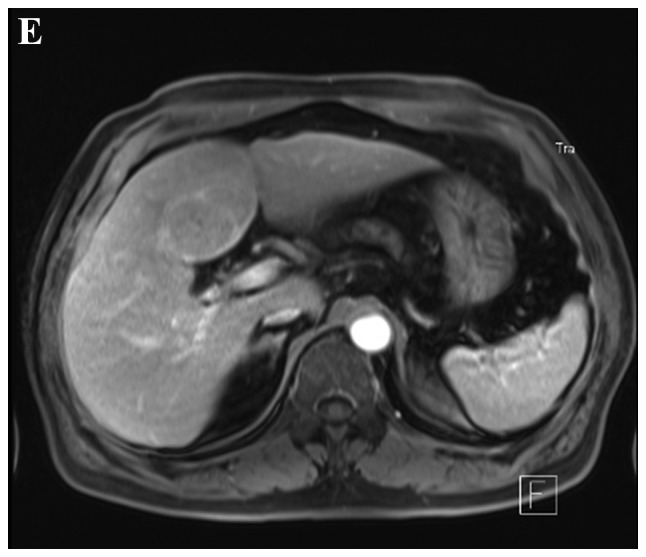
MRI images of the lesion. The solitary lesion at the fourth hepatic segment is (A) hypointense on T1WI and (B) hyperintense on T2WI. The dynamic gadolinium-diethylenetriaminepentaacetic acid MRI protocol showing a (C) mild ring-like enhancement during arterial phase, (D) continued and prominent enhancement in portal venous phase, and (E) that the enhancement of the tumor has decreased in the delayed phase, and that the septum is enhanced. MRI, magnetic resonance imaging; WI, weighted image.
